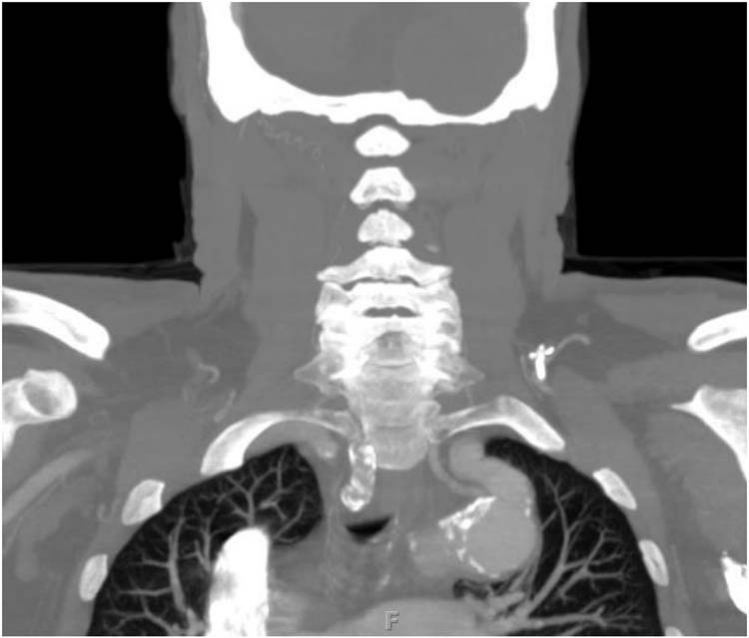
Preoperative CTA demonstrating aberrant left vertebral artery originating from the aortic arch, just proximal to the LSA.
